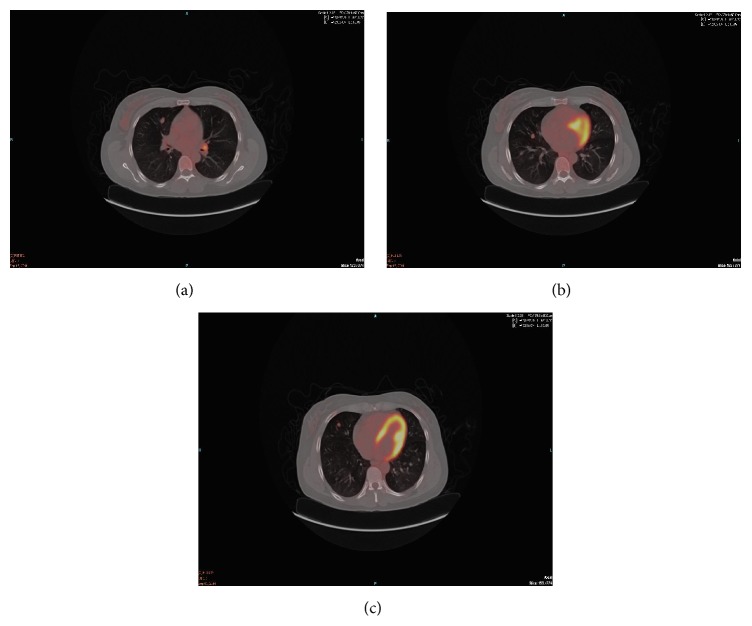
Multidisciplinary review of PET CT.
